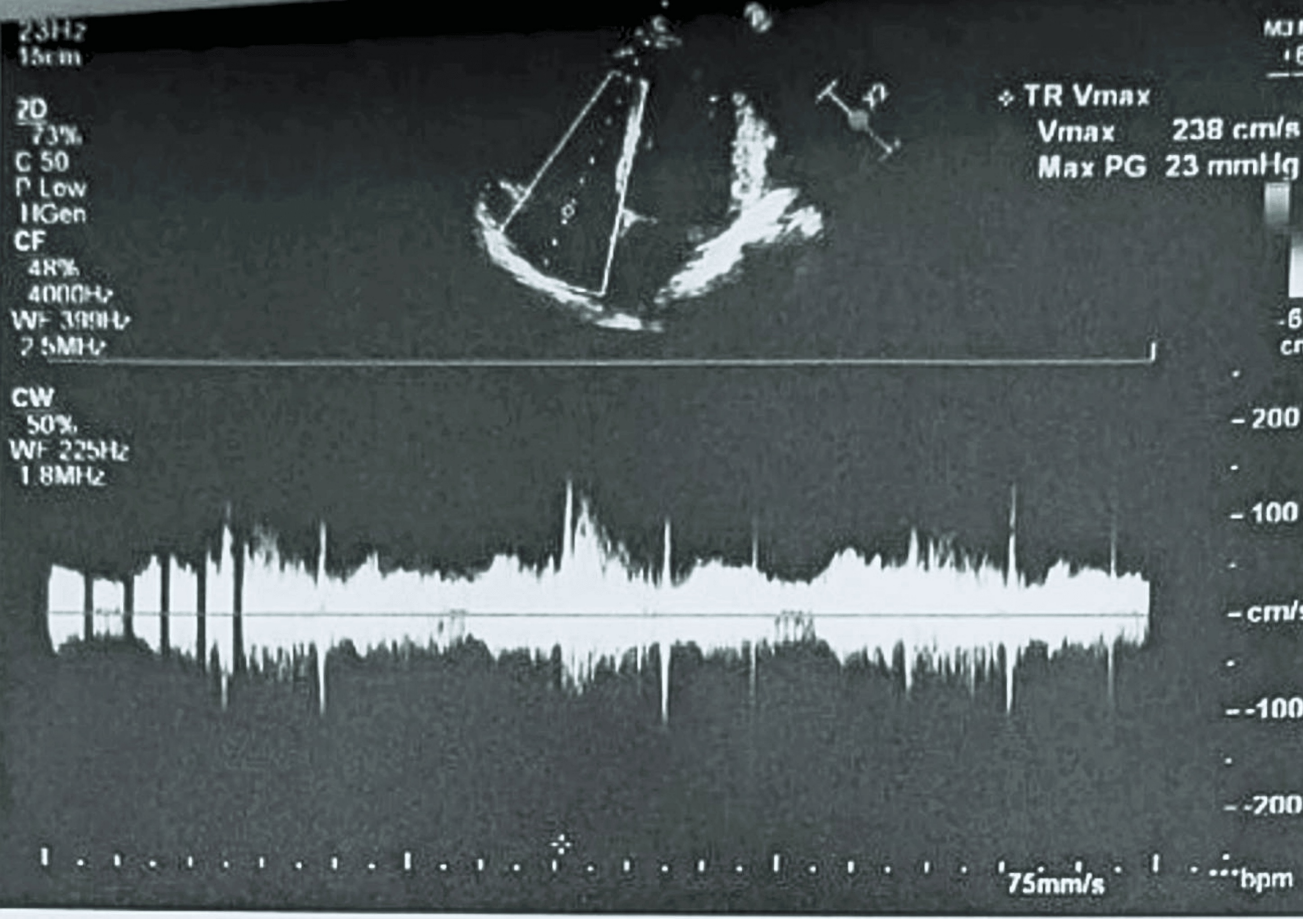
Echocardiography showing systolic regurgitant flow across the tricuspid valve probably due to myocarditis
